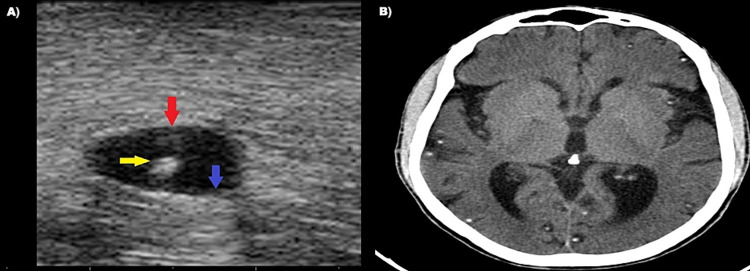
(A) Point-of-care soft tissue ultrasound of neck swellings showing cystic lesion (red arrow) with a hyperechoic focus (scolex - yellow arrow) and internal debris (blue arrow) suggesting soft tissue cysticercosis. (B) Computed tomography of the brain showing multiple cysticercoid granuloma with perilesional edema and calcification suggesting neurocysticercosis.
